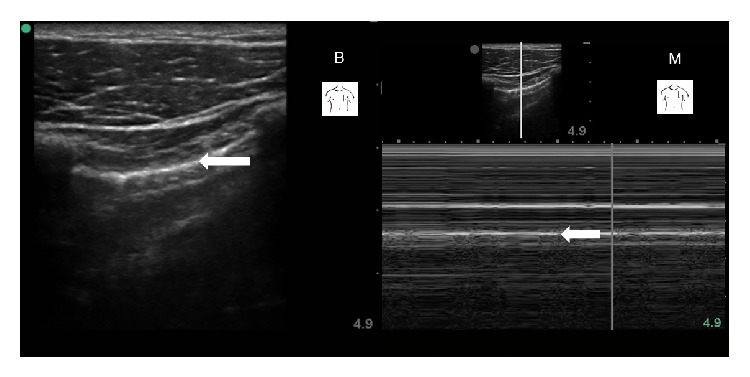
Healthy volunteer for comparison: B-mode and M-mode sonography using a linear transducer positioned in the mid-clavicular line at the 3rd intercostal space. Arrows indicate the pleural lines (B: B-mode, M: M-mode).
